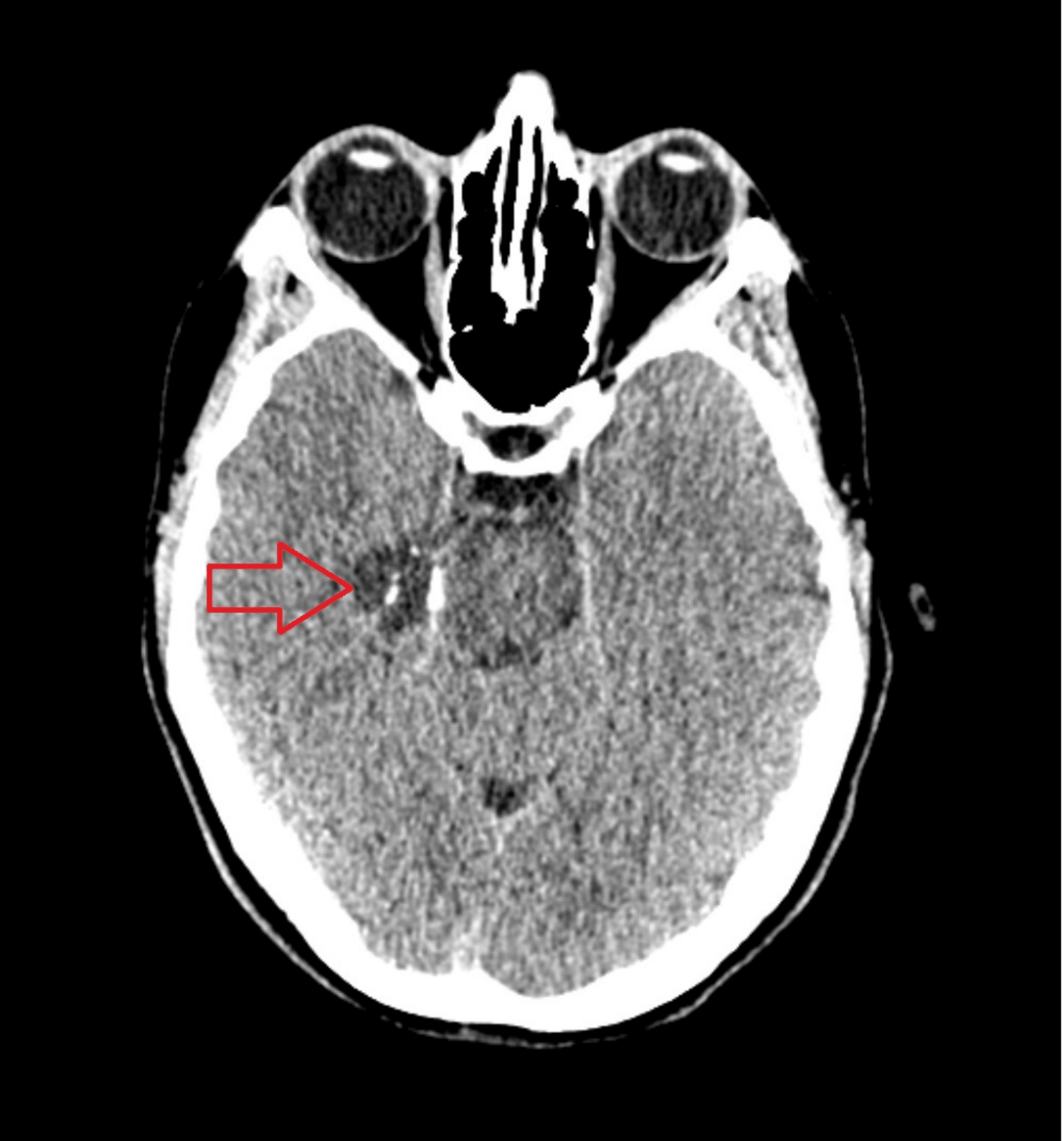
CT showing a newly identified suspicious cyst not previously described
